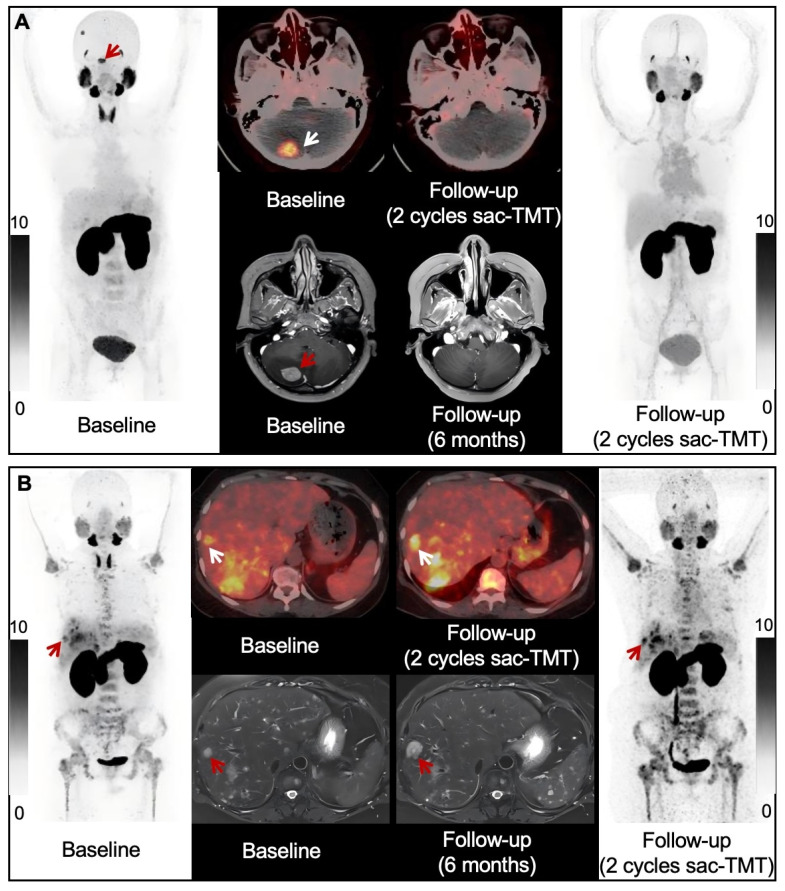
Changes in ^68^Ga-MY6349 uptake as a potential predictor of response to Trop2-ADC. (A) A 43-year-old woman with metastatic TNBC. Compared with the baseline scan, follow-up ^68^Ga-MY6349 PET/CT (two cycles after sac-TMT treatment) revealed significant decrease in ^68^Ga-MY6349 uptake in the brain metastases. The follow-up MRI (6 months after treatment) revealed complete response, with disappearance of the brain lesions. (B) A 46-year-old woman with metastatic TNBC. Compared to the baseline scan, follow-up ^68^Ga-MY6349 PET/CT (two cycles after sac-TMT treatment) revealed higher ^68^Ga-MY6349 uptake in most liver and bone metastases. The follow-up MRI (6 months after treatment) revealed progressive disease. ADC: antibody-drug conjugate; CT: computed tomography; MRI: magnetic resonance imaging; PET: positron emission tomography; sac-TMT: sacituzumab tirumotecan; TNBC: triple-negative breast cancer; Trop2: trophoblast cell-surface antigen 2.
